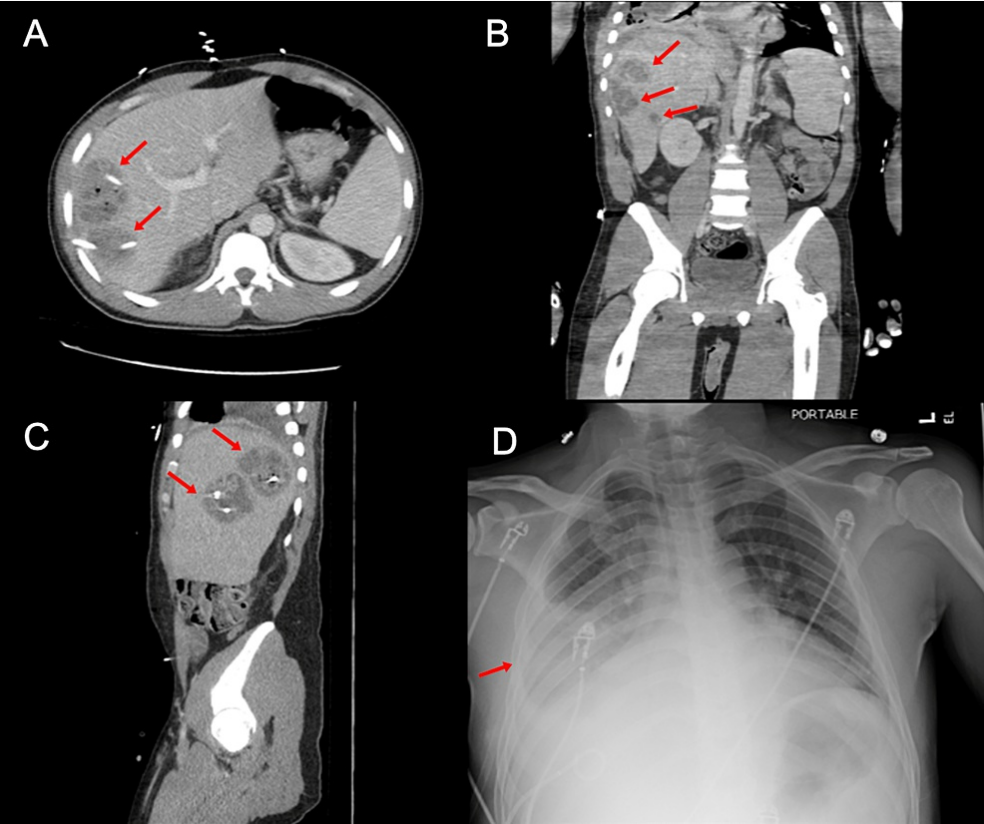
Transverse (A), coronal (B), and sagittal (B) anatomic projections of contrast CT abdomen and pelvis demonstrating three complex cystic hepatic lesions (red arrows); (D) Chest X-ray anteroposterior view, showing interstitial worsening in the aeration of the right lower lobe associated with increase in the right pleural effusion (red arrow)
